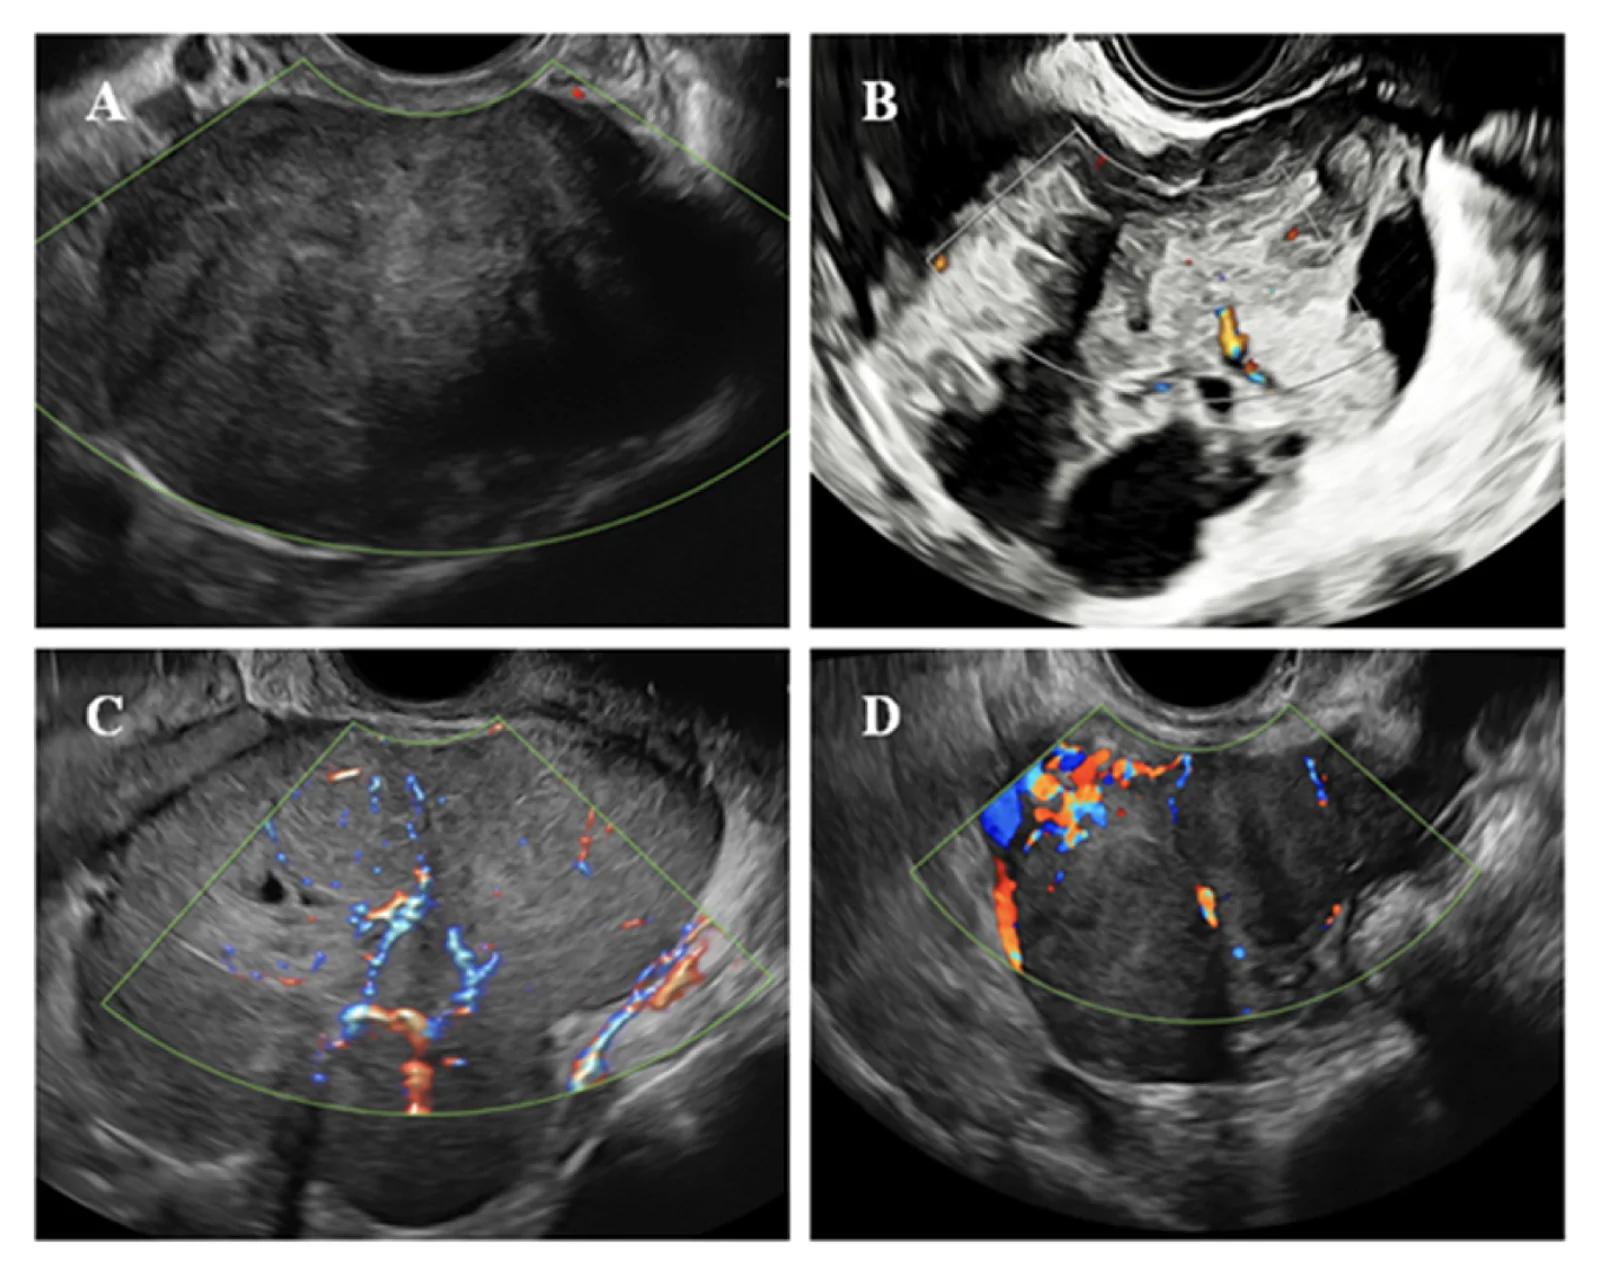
Ultrasound images showing adnexal mass vascularization, according to IOTA terms and definitions: color score 1 when no blood flow is detected (A), color score 2 when minimal flow is detected (B), color score 3 when moderate flow is detected (C), color score 4 when intense flow is detected (D).
